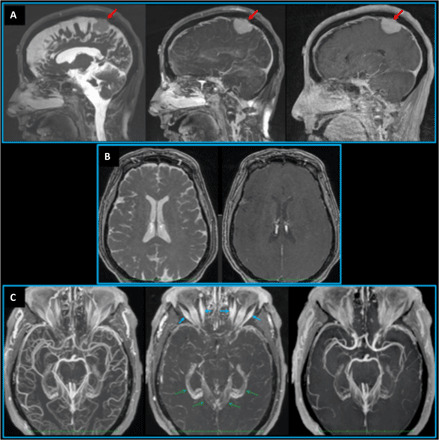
T_1_RESS sequence comparisons in different patients. (A) Patient with a meningioma (red arrows). Left: Unmodified three-dimensional (3D) bSSFP acquisition shows bright signal from cerebrospinal fluid and poor tissue contrast, precluding diagnostic evaluation of the tumor or blood vessels. Middle: With bT_1_RESS, cerebrospinal fluid and healthy brain tissue appear relatively dark. There is excellent visibility of the contrast-enhancing meningioma, nasal mucosa, venous sinuses, and their tributaries. Right: With 3D spoiled GRE, tumor visibility and vessel detail are inferior to bT_1_RESS. (B) Patient with unremarkable brain MRI. Comparison of axial multiplanar reconstructions from uT1RESS using CMα flip angles of 0° (left) and 75° (right). As with bT_1_RESS, without the effect of a CMα RF pulse, there is poor visualization of contrast-enhancing tissues (e.g., choroid plexuses). (C) Axial maximum intensity projections (24 mm thick) from a patient with an unremarkable brain MRI. Left: bT_1_RESS. Middle: uT_1_RESS. Right: 3D spoiled GRE. bT_1_RESS shows much more vascular detail than 3D spoiled GRE, while uT_1_RESS shows extensive suppression of intravascular signals. Because the signals from blood vessels and nonenhancing background tissues are suppressed, contrast enhancement of the extraocular muscles (blue arrows), dura, and choroid plexuses (green dashed arrows) is better shown by uT_1_RESS than by the other imaging techniques.
